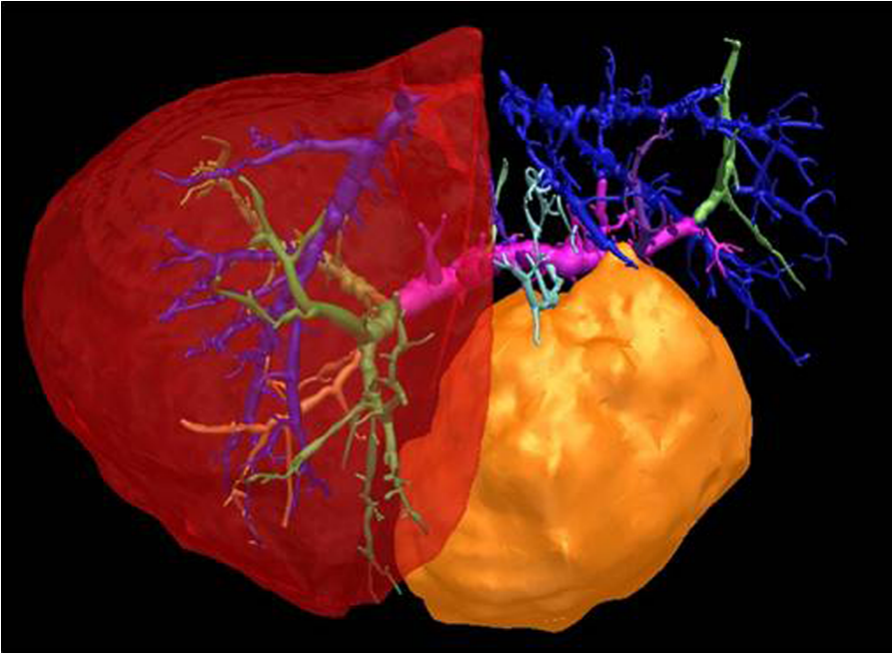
Three-dimensional computation from a preoperative CT scan of the patient with a large liver metastasis (superior view). The portal vein is pink and green, the hepatic veins are blue, and the tumor is yellow.
